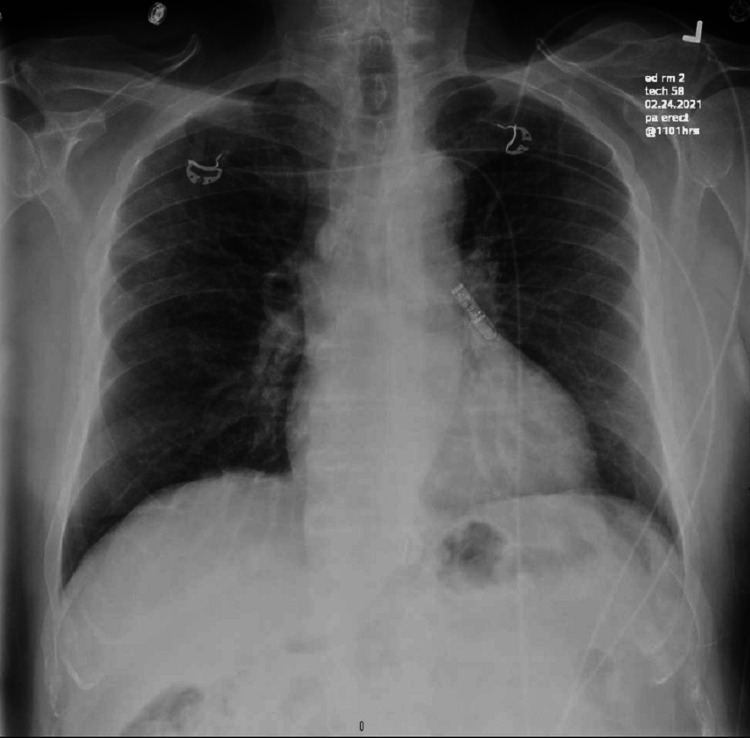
Chest X-ray showing ill-defined opacities over bilateral upper lobe with no consolidation.
